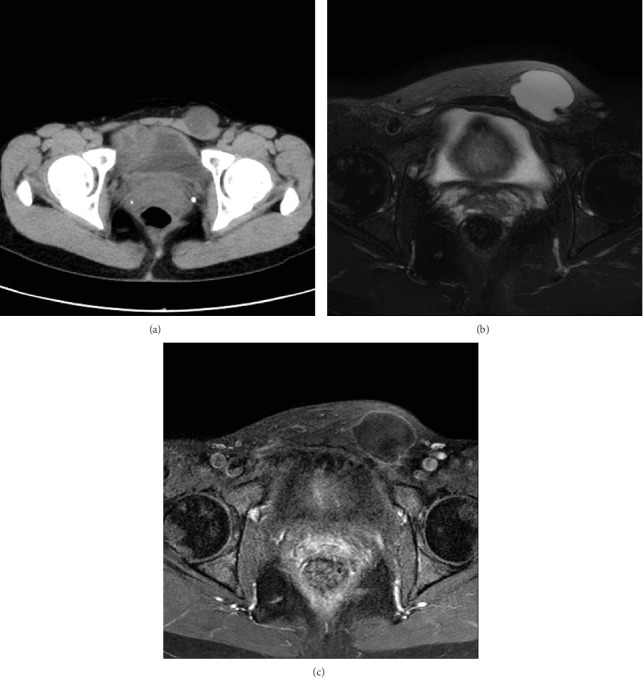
(a) CT displaying a 30 mm low-density mass in the left inguinal region. (b) Fat-suppressed T2-weighted MRI displaying a subcutaneous mass with homogeneous high-intensity signal. (c) Gadolinium-enhanced fat-suppressed T1-weighted MRI revealing a well-circumscribed cystic mass with a thin wall.
